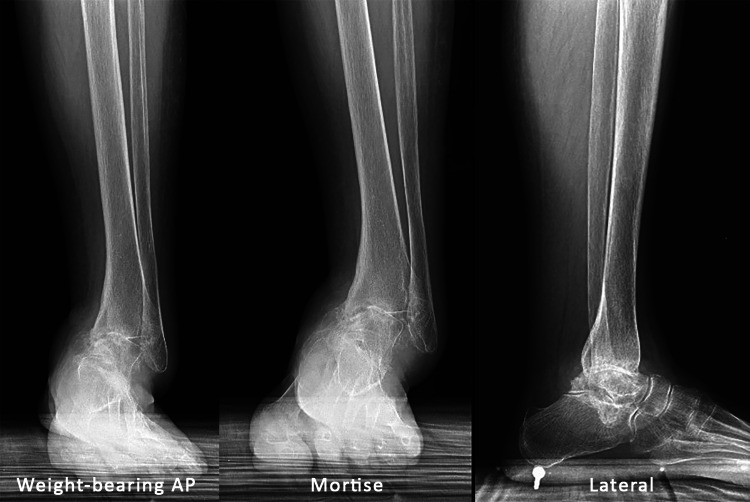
Left ankle: AP, mortise, and lateral radiographs Weight-bearing anteroposterior (AP), mortise, and lateral of the left ankle demonstrating significant tibiotalar and subtalar osteoarthritis with varus hindfoot and talar tilt.
